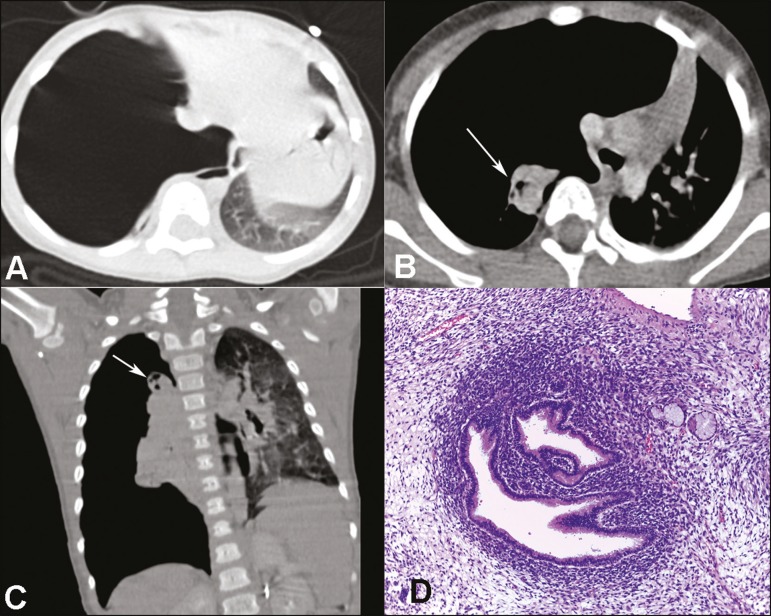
CT scan of the chest showing a voluminous pneumothorax on the right
